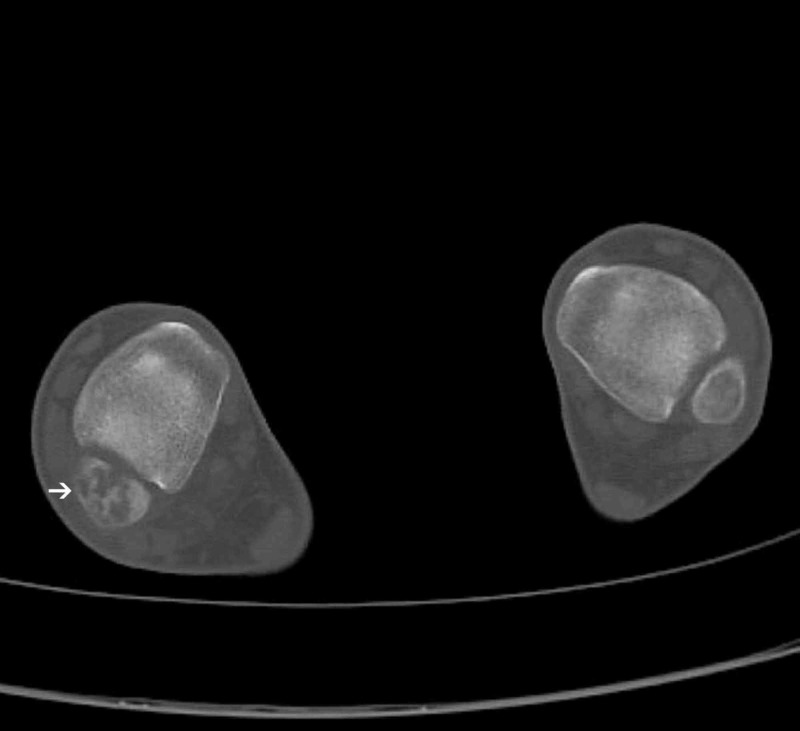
CT scan of the right ankle joint Osteolysis of the right fibula with thinning of the bone cortex on its medial side (white arrow)
